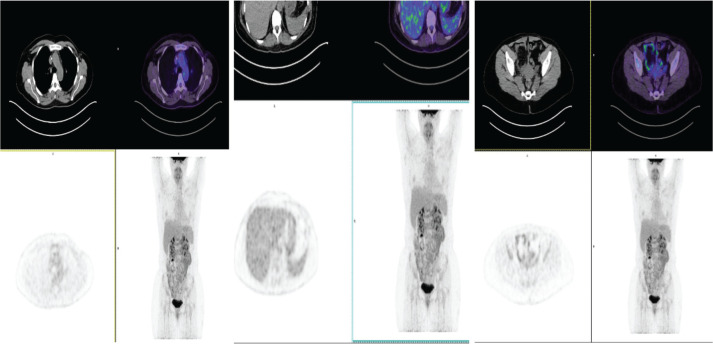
PET-CT of June 2022: no evidence of metabolic changes suggesting local or distant tumour viability. Resolution of oligometastases in T11.
